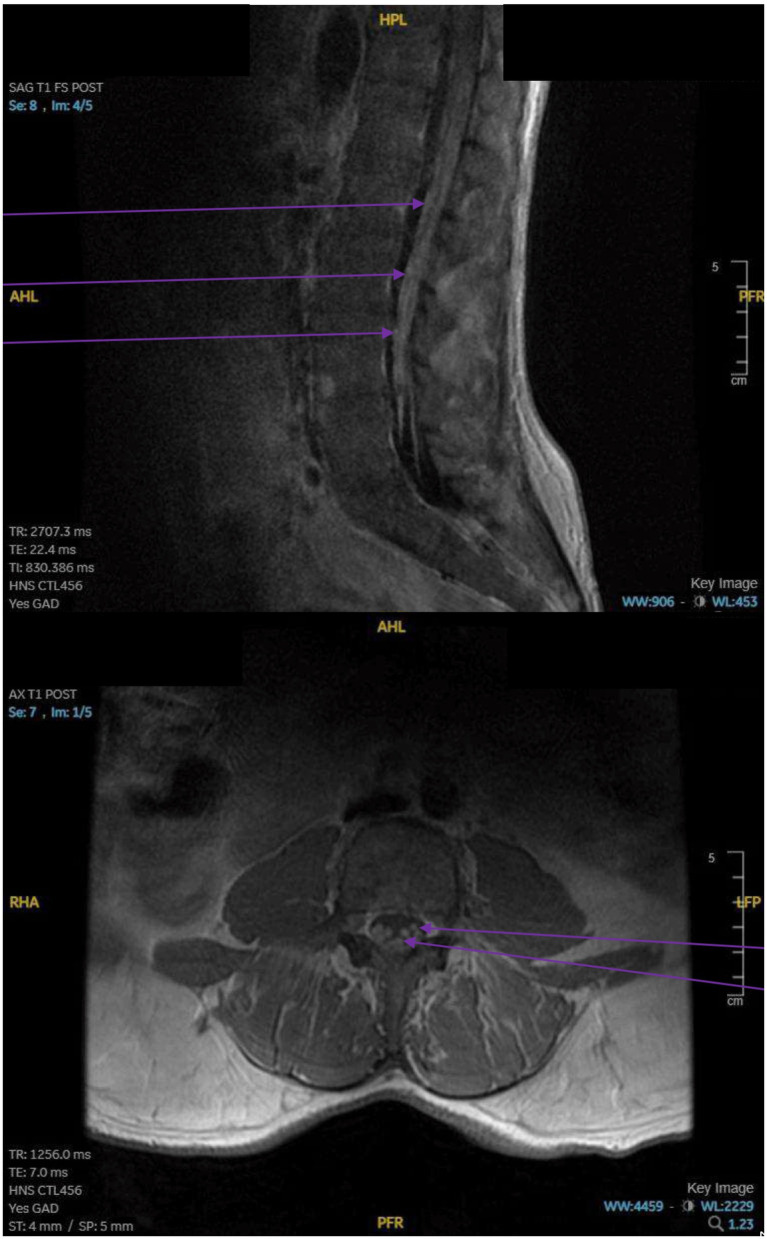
Saggital and Axial MRI of Lumbar Spine, showing distinct nodular metastasis (Purple Arrow).
